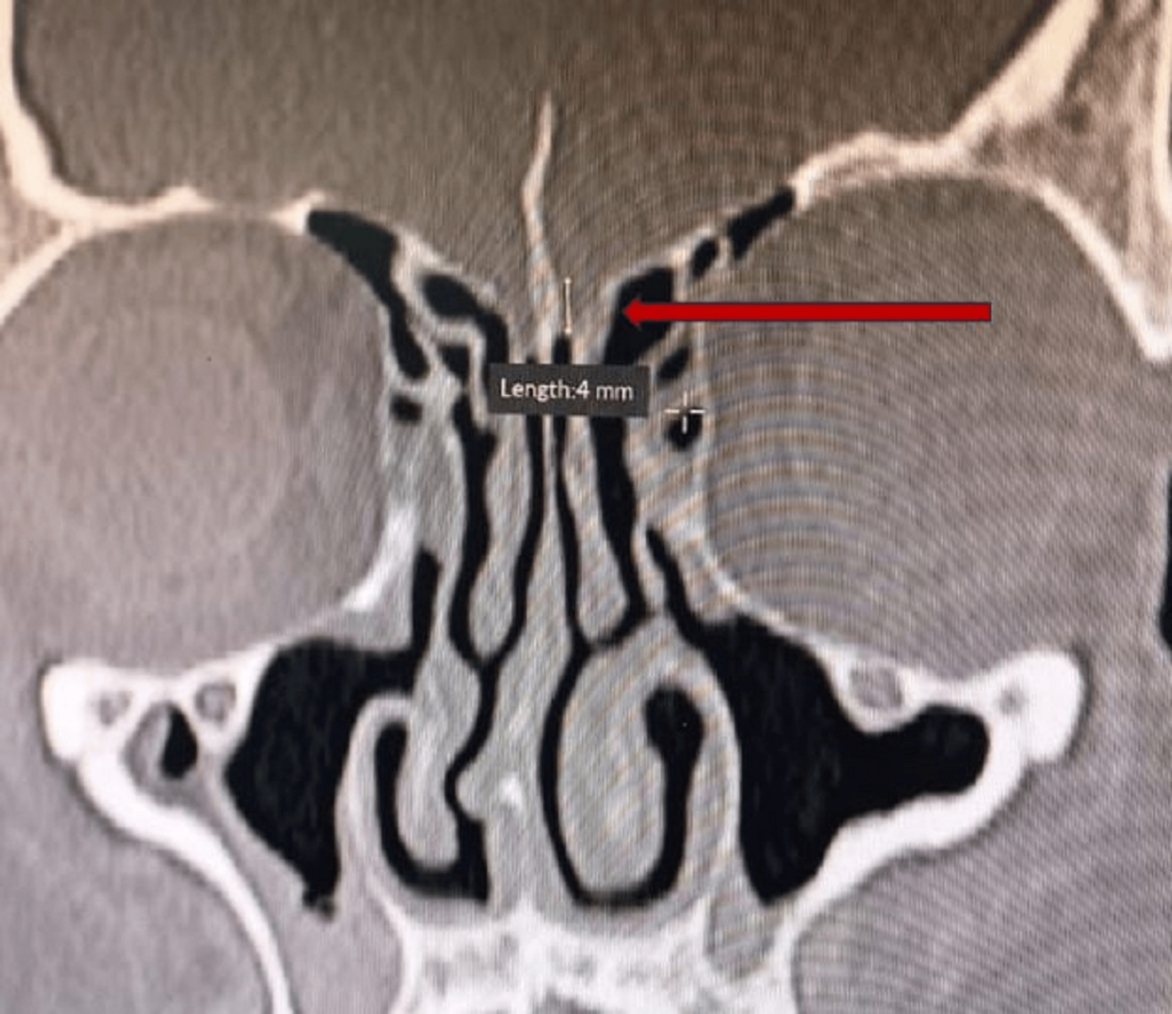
Coronal section of CT scan paranasal sinuses showing left sided type 2 Keros
